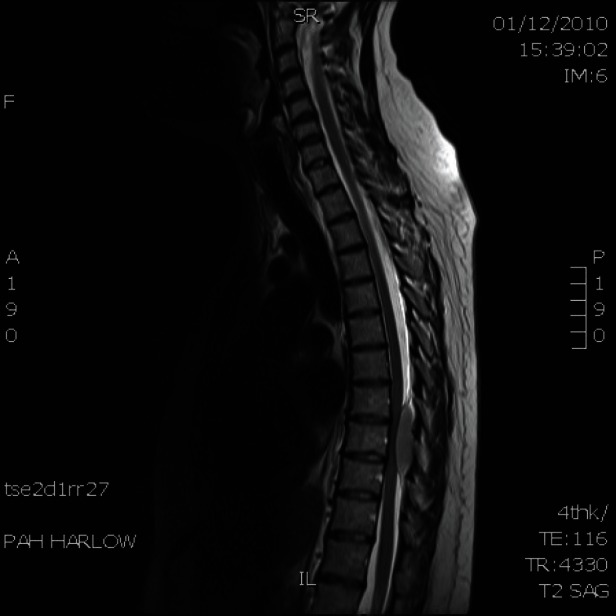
MRI scan of spine shows soft tissue mass posterior to T9 causing moderate cord compression.
